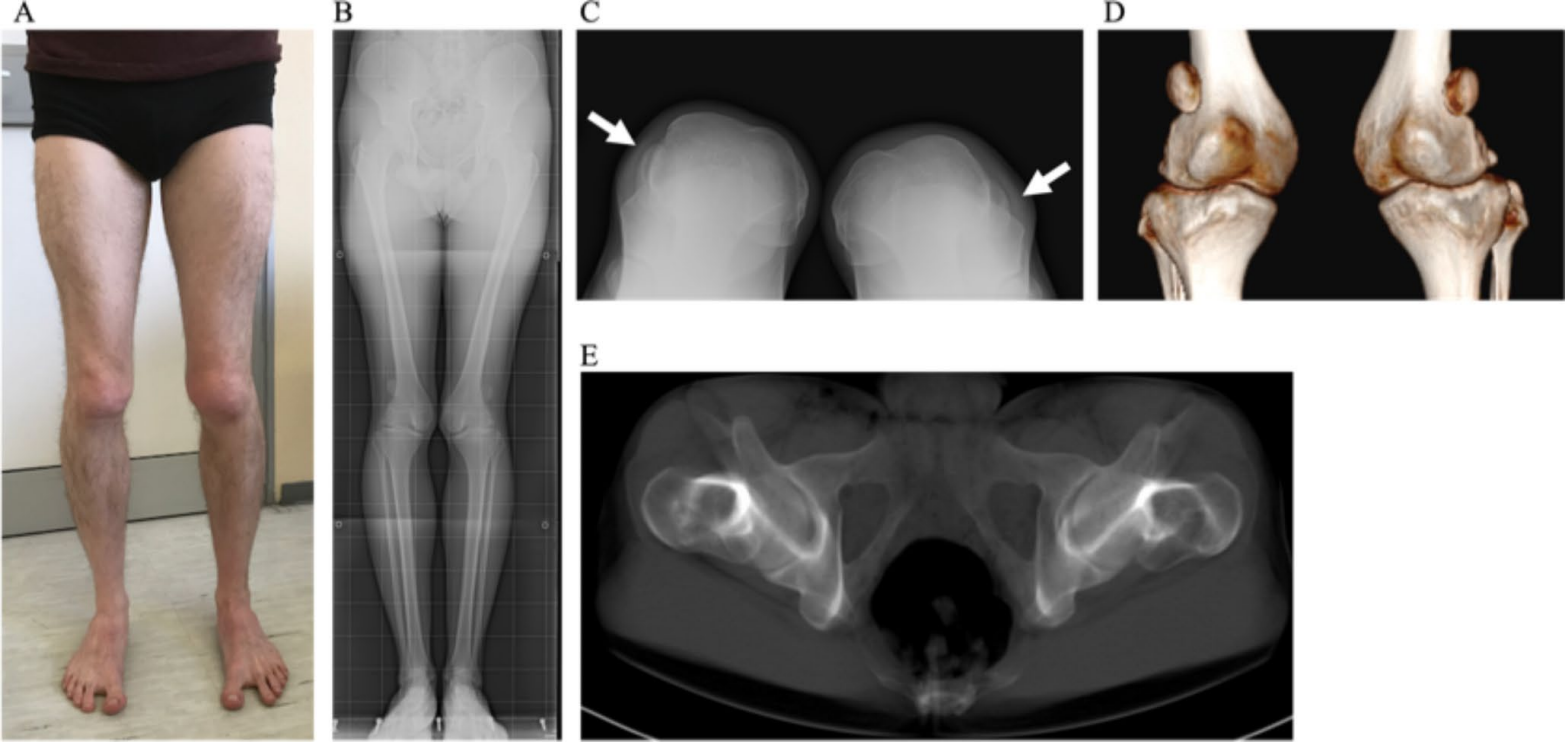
Clinical picture of the proband before surgery. A clinical aspect; B X-ray images in standing position that show internal torsion of the knees, with femoral valgus and tibial varus and dislocated patella; C sunrise view X-ray showing complete lateral dislocation of the hypoplastic patella and flat femoral trochlea on both sides (arrows); D 3D CT scan image of both dislocated patellae; (E) the retroversion of femoral neck seen in CT scan reconstruction
